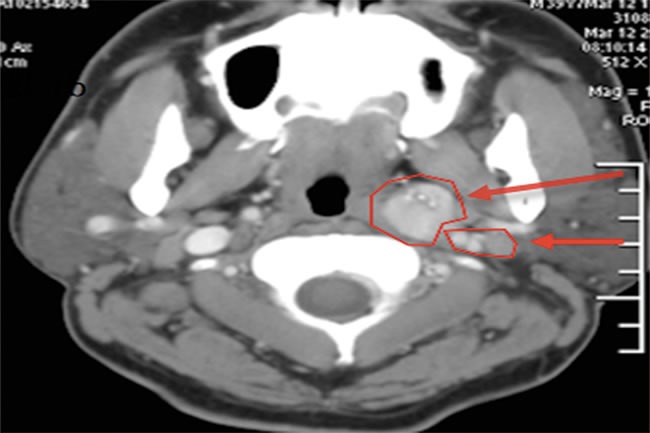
Preoperative CT scan of the neck Left thyroid mass (long arrow). Multiple enhanced lymph nodes were found in the periphery of the thyroid gland and in the left carotid sheath (short arrow).
